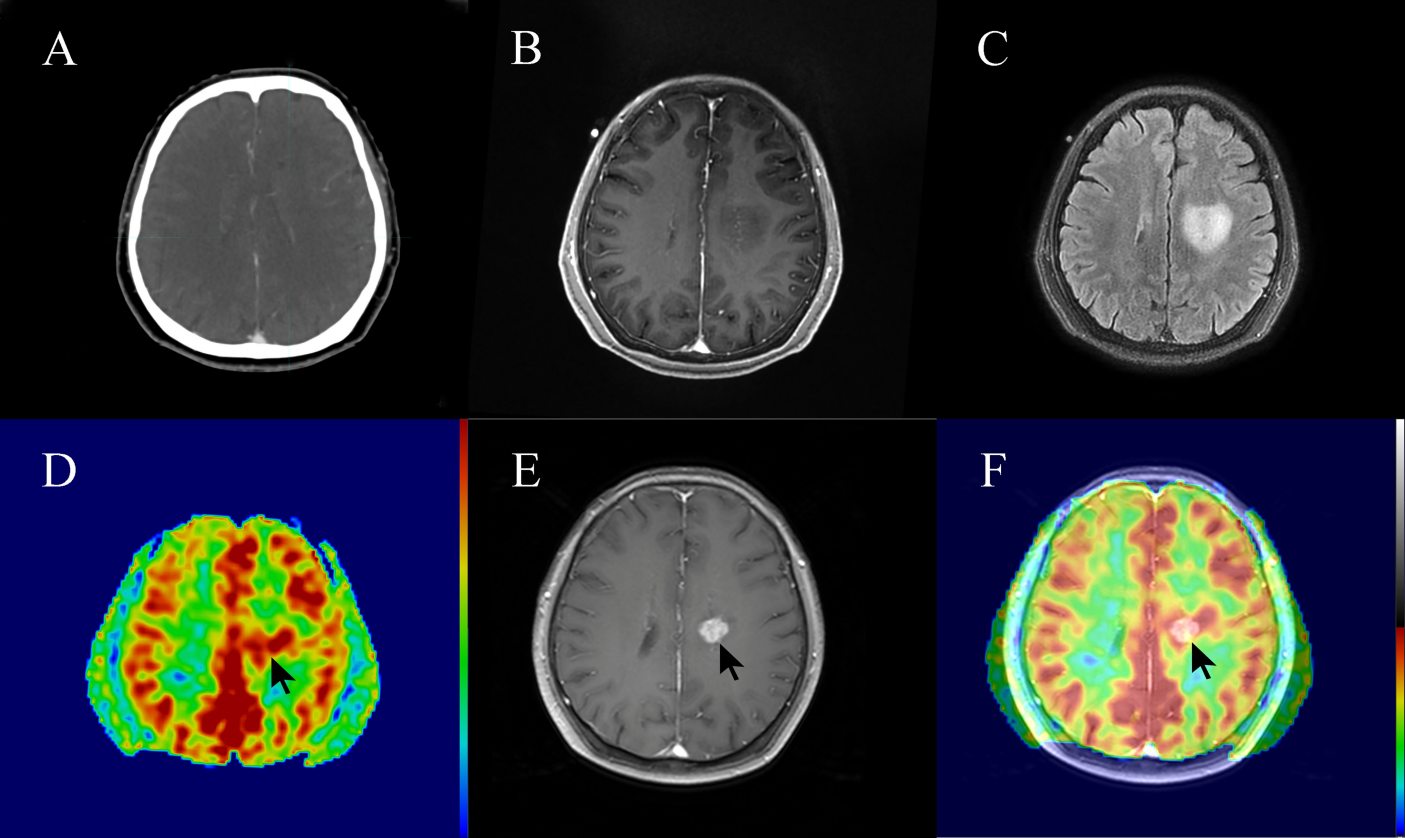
A 62-year-old patient with non-enhancing low-grade glioma (WHO grade II astrocytoma) showed focal post-radiotherapy contrast enhancement within a prior non-enhanced and high-perfusion area at 3 months following radiotherapy. The patient had clinical symptoms such as weakness of the right lower limb, considering the tumor progression. (A) Planning contrast-enhanced CT (CE-CT) image. (B) Contrast-enhanced T1-weighted imaging (CE-T1WI) before radiotherapy. (C) T2 fluid-attenuated inversion recovery (T2 Flair) image before radiotherapy. (D) three-dimensional arterial spin labeling (3D-ASL) image before radiotherapy. (E) CE-T1WI at 3 months following radiotherapy. (F) Fusion image of (D, E).
